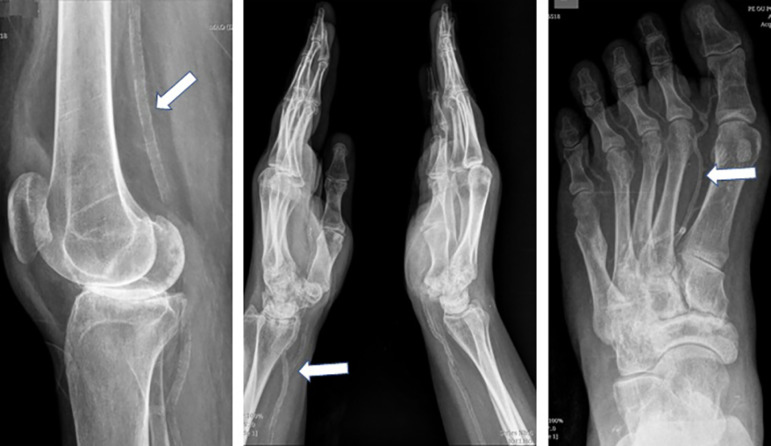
Simple X-Rays showing extensive calcification in the arteries (white
